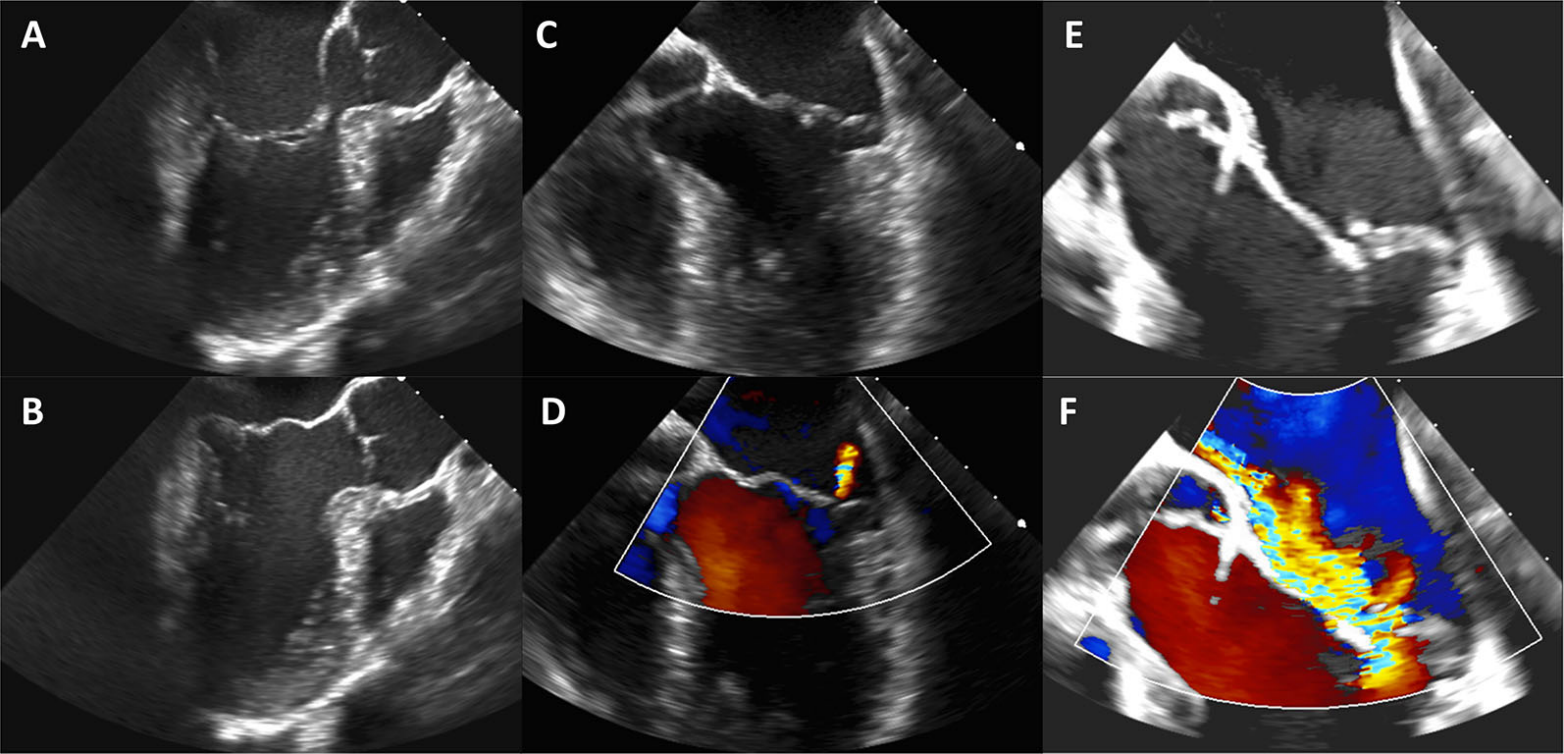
Transoesophageal echocardiographic findings. A, B Long-axis view in transesophageal echocardiogram (TOE) before antibiotic treatment in diastole (A) and systole (B) showing a billowing of the mitral valve, a finding already seen in a transthoracic echocardiocardiography (TTE) performed 3 days earlier. There were no evidence of vegetation or perivalvular abscess of the mitral valve. Moreover, no evidence of endocarditis of the submitral apparatus were seen in TTE as well as TOE. All other valves were normal. C, D Long-axis view in TOE (C) and the corresponding Color Flow Doppler (D) showing a mild mitral regurgitation. E, F Long-axis view in TOE (E) and the corresponding Color Flow Doppler (F) 6 weeks after completion of the antibiotic treatment demonstrating a severe eccentric mitral valve regurgitation with P2 prolaps secundary to chordea rupture. No vegetation or abscess were detected. Hemocultures were sterile and laboratory tests did not reveal any elevated inflammatory parameters (WBC 0.8 G/L; CRP < 1 mg/L). It is recognized that identification of vegetation using echocardiography may be difficult after recent embolization as it was the case in this patient. Since baseline TOE demonstrated only mild billowing of the posterior mitral leaflet, a spontaneous degenerative chordal rupture in a young patient would appear very unlikely in this situation, supporting our hypothesis that an infectious process limited to the sub-valvular apparatus led to the chordal rupture. Moreover, surgical inspection confirmed the isolated chordal rupture without damage of the mitral valve leaflet, also correlating with previous ^18^F-FDG PET/CT finding
